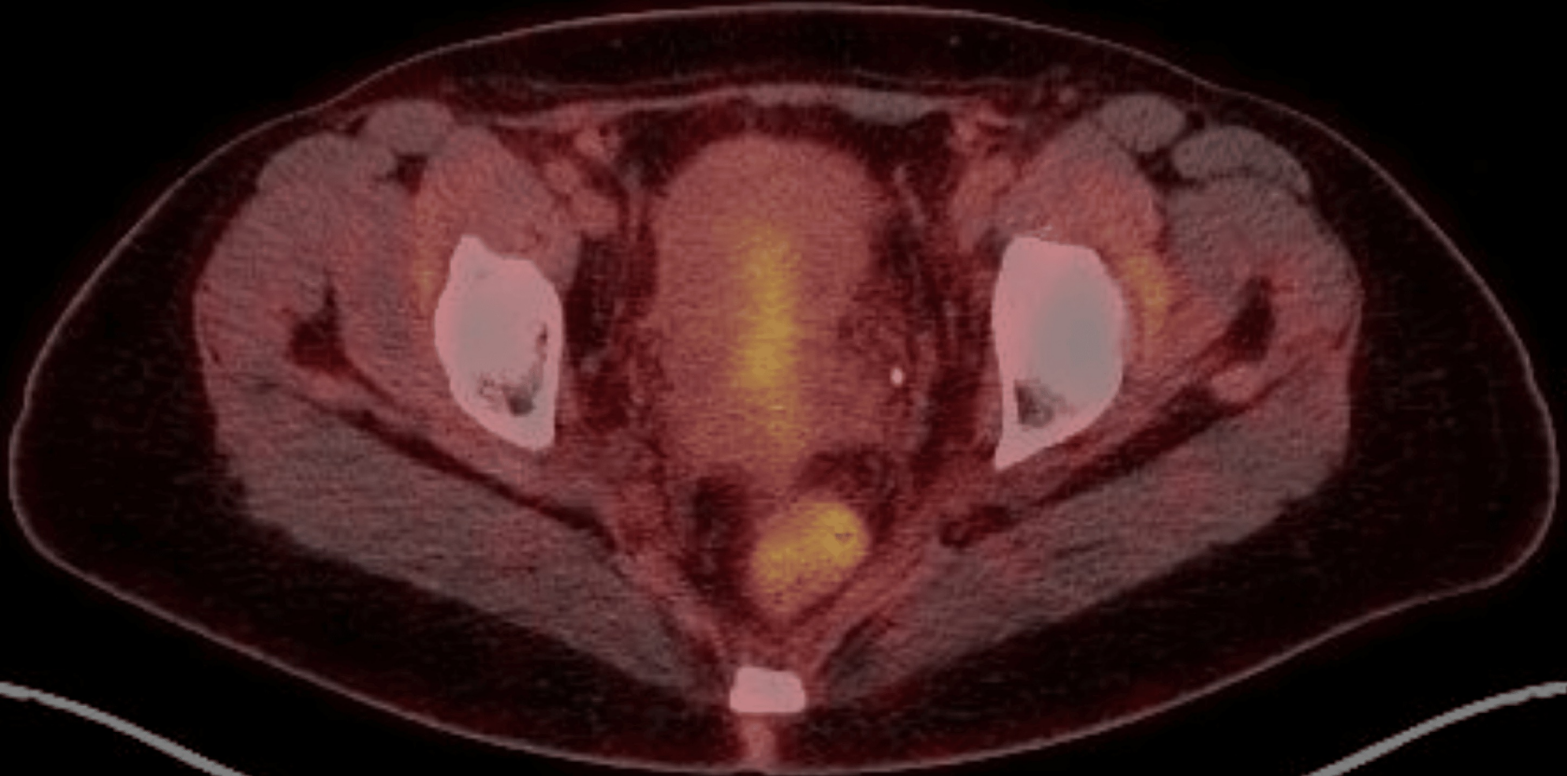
FDG PET-CT demonstrates significant regression of the cervical mass post-CCRT with regard to size and avidity as well as the left external iliac lymph node. FGD: fluorodeoxyglucose; PET-CT: positron emission tomography-computed tomography; CCRT: concurrent chemo-radiation
